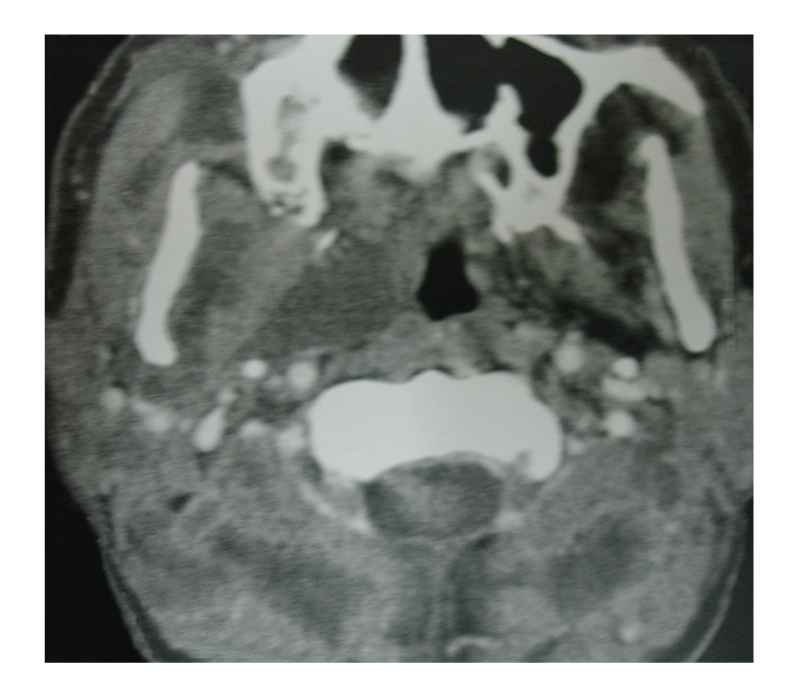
Neck CT scan of the case shows multiple cystic lesions in the neck and right parapharyngeal space.
